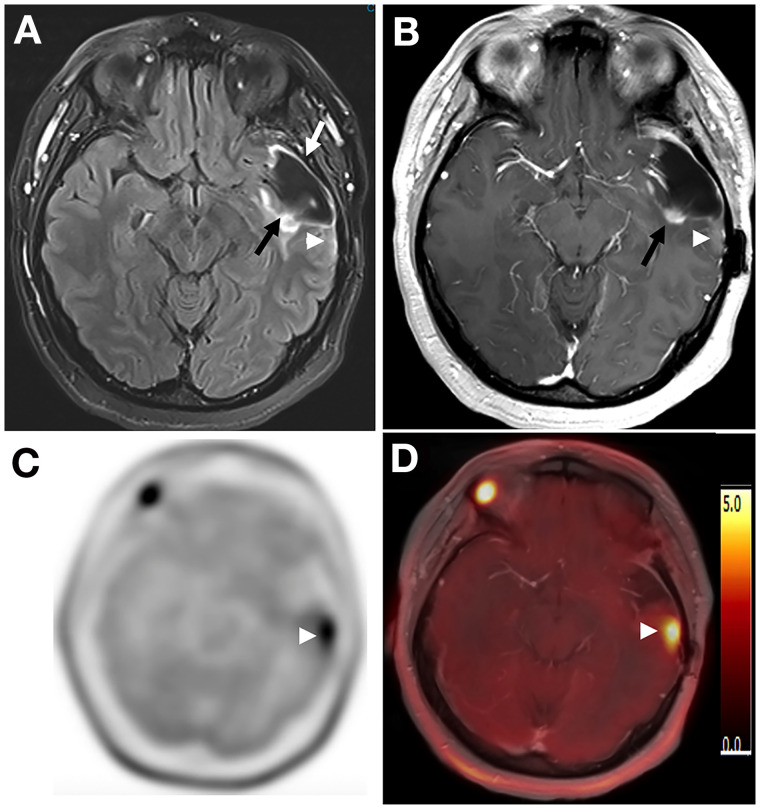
(A) Axial T2-weighted FLAIR image through level of midbrain shows large cystic resection cavity in left temporal lobe (white arrow). There is ill-defined T2 abnormality at medial aspect of resection cavity (black arrow). No obvious abnormality is noted posterior and lateral to resection cavity (arrowhead). (B) Axial postcontrast T1-weighted image through same level better shows focal area of contrast enhancement (arrow). This enhancing focus has been followed up since prior treatment. Subtle contrast enhancement, new finding compared with previous MRIs, is noted posterior and lateral to resection cavity (arrowhead). (C) Axial reconstruction of ^11^C-methionine PET images through same level shows intense ^11^C-methionine uptake posterior and lateral to resection cavity (arrowhead) corresponding to new subtle T1 enhancement. (D) Postcontrast T1-weighted ^11^C-methionine PET/MRI image also shows that ^11^C-methionine abnormality corresponds to new subtle enhancement at posterior and lateral aspect of resection cavity (arrowhead).
